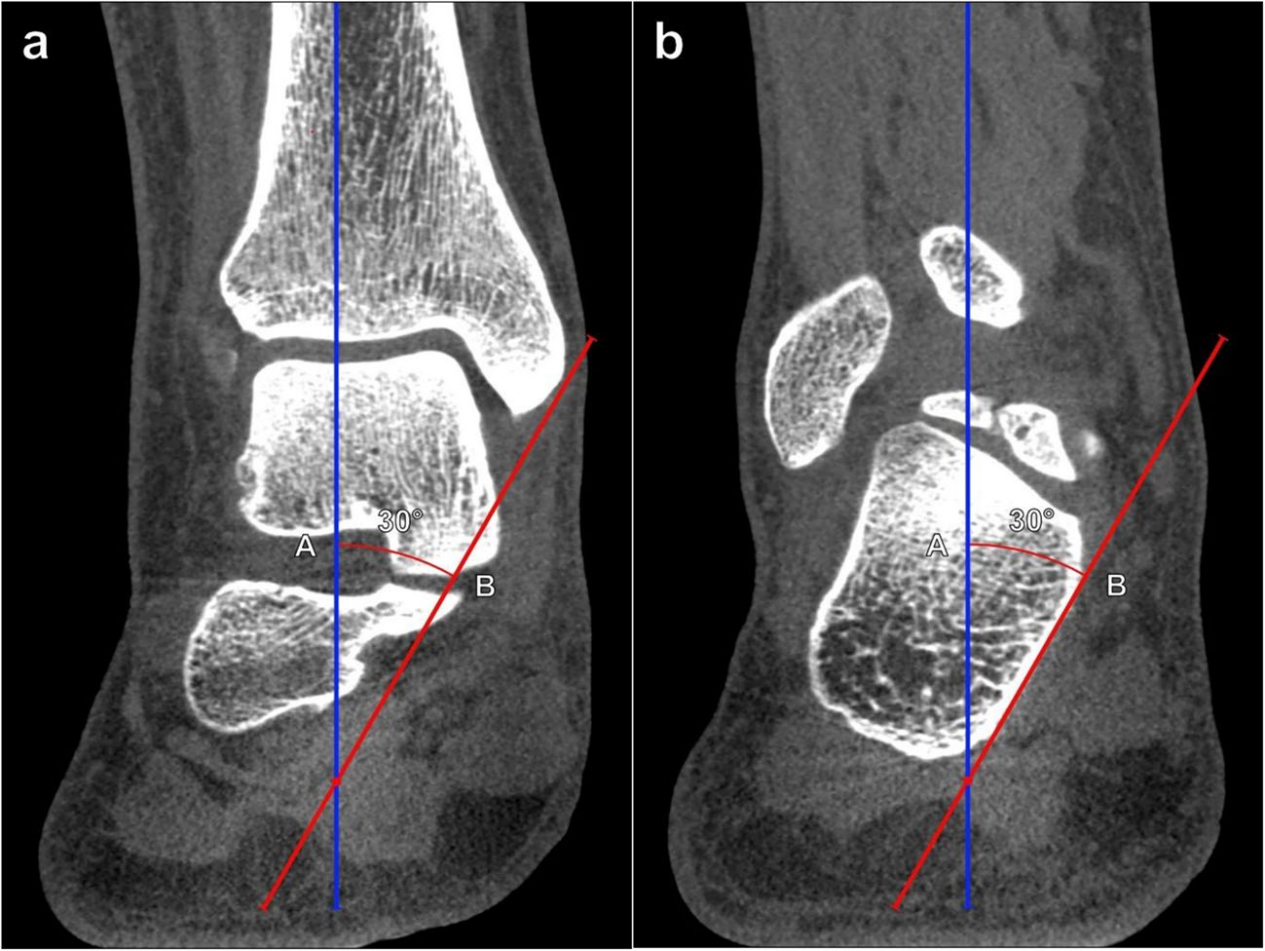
Tibiocalcaneal (hindfoot valgus) angle on coronal reformatted cone beam CT (CBCT) of the ankle: CBCT images are shown at the level of the distal tibia (a), which depicts the tibial axis (A, blue line) and at the level of the calcaneus (b), which shows the appropriate reference for the medial calcaneal cortex (B, red line). The angle is formed between lines drawn along the axis of the distal tibia (A) and tangent to the medial border of the calcaneus (B). Please note that the reference lines are drawn on two separate coronal reformatted images when using CBCT images and need to be superimposed to determine the angle. The hindfoot valgus angle normally measures ≤ 6° utilizing weightbearing radiography or CT, and larger angles indicate greater degrees of hindfoot valgus, which is associated with loss of the medial midfoot arch and posterior tibialis tendon dysfunction
